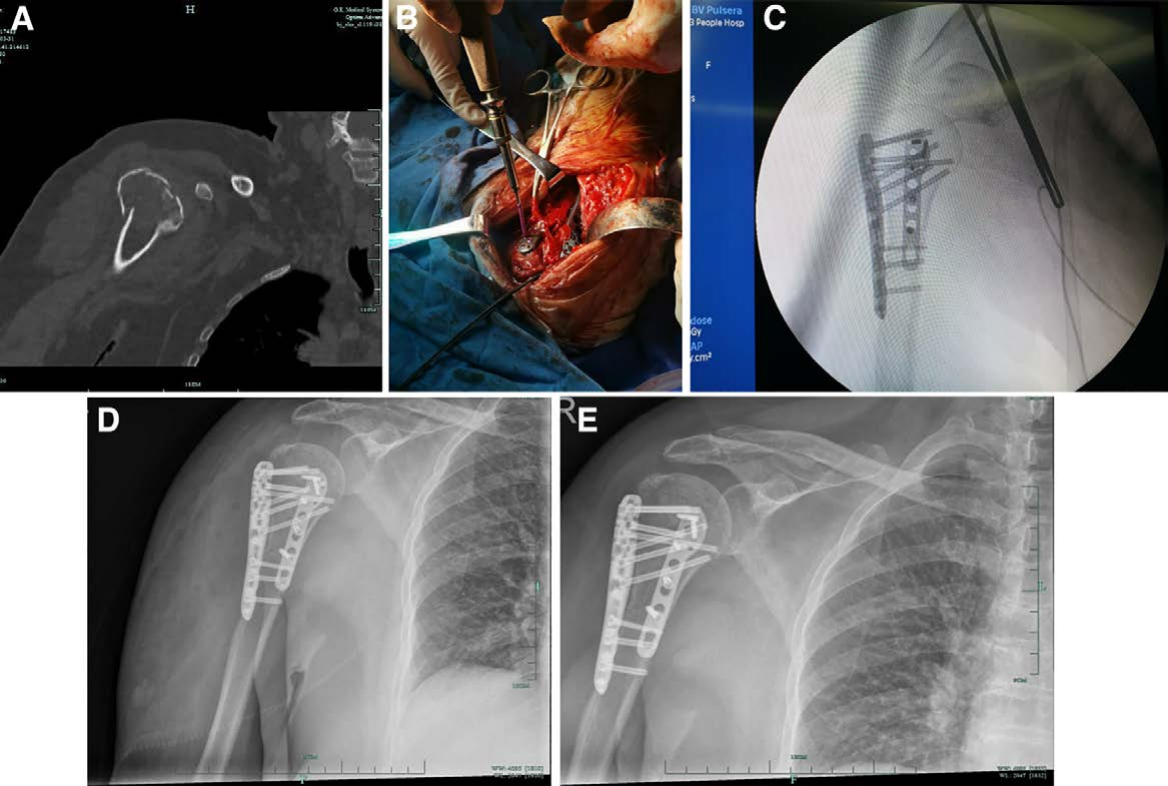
Typical case 1. (A) Preoperative computed tomography of the shoulder revealed a 70-year-old woman with comminuted and displaced fracture of the right proximal humerus. (B) Intraoperative image: after satisfactory reduction of the comminuted fracture of the proximal humerus, fixation with a proximal humerus locking plate was performed 0.5 to 1 cm below the greater tubercle of the humerus. Then, the anterior medial humerus was exposed after the upper limb abduction and external rotation, the antero-medial support plate was inserted medial to the intertubercular groove, and the screws were inserted. (C) Intraoperative X-ray image: Anteromedial support plate combined with lateral locking compression plate fixed the proximal humerus fracture, the fracture reduction was satisfactory, and the internal fixation position were good. (D) Postoperative shoulder joint X-ray: the reduction of the proximal humeral fracture was satisfactory, the position of the anteromedial support plate and lateral locking compression plate were good, and the length of the screws were good. (E) Six months after surgery shoulder joint X-ray: the proximal humerus fracture healed. There was no necrosis or varus deformity of the humerus. The position of the double plates were good, and there were no loosening or breakage of the screws.
